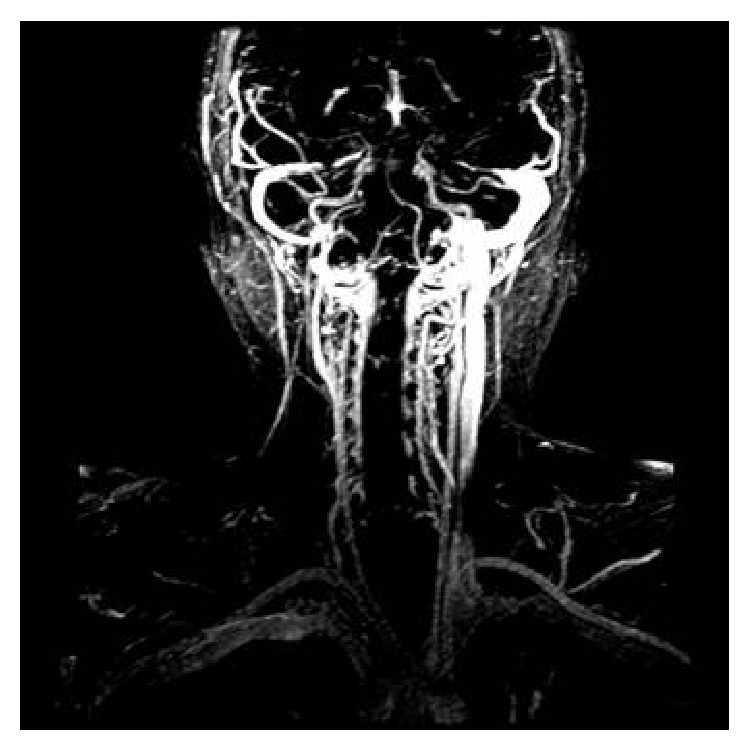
Sagittal view of collateral system (dynamic magnetic resonance image).
